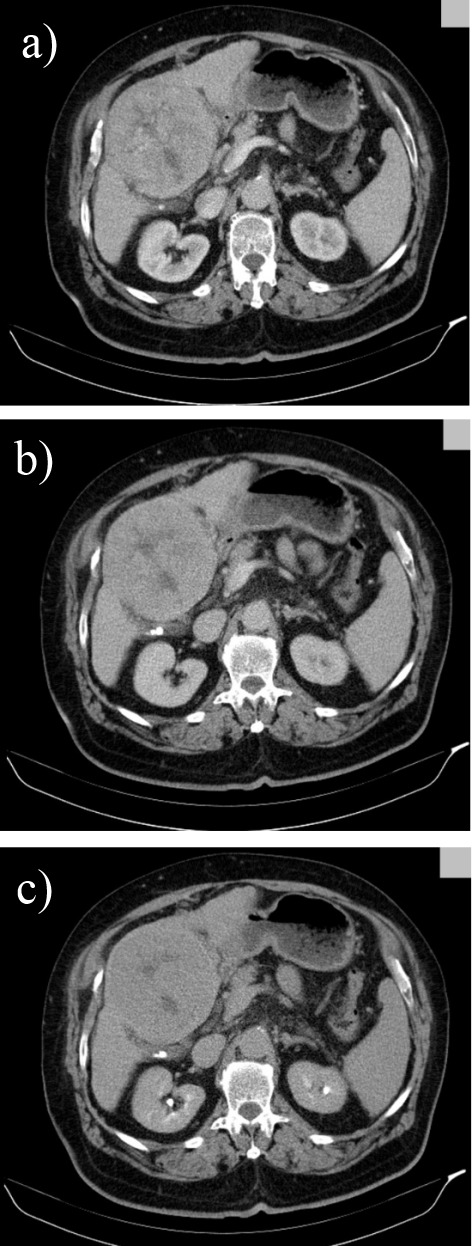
Arterial (a), portal (b) and late phase (c) 16 slices CT in a cirrhotic patient revealing a well-differentiated hepatocellular carcinoma in segment IV with extracapsular extension, heterogenous contrast uptake and central necrosis
